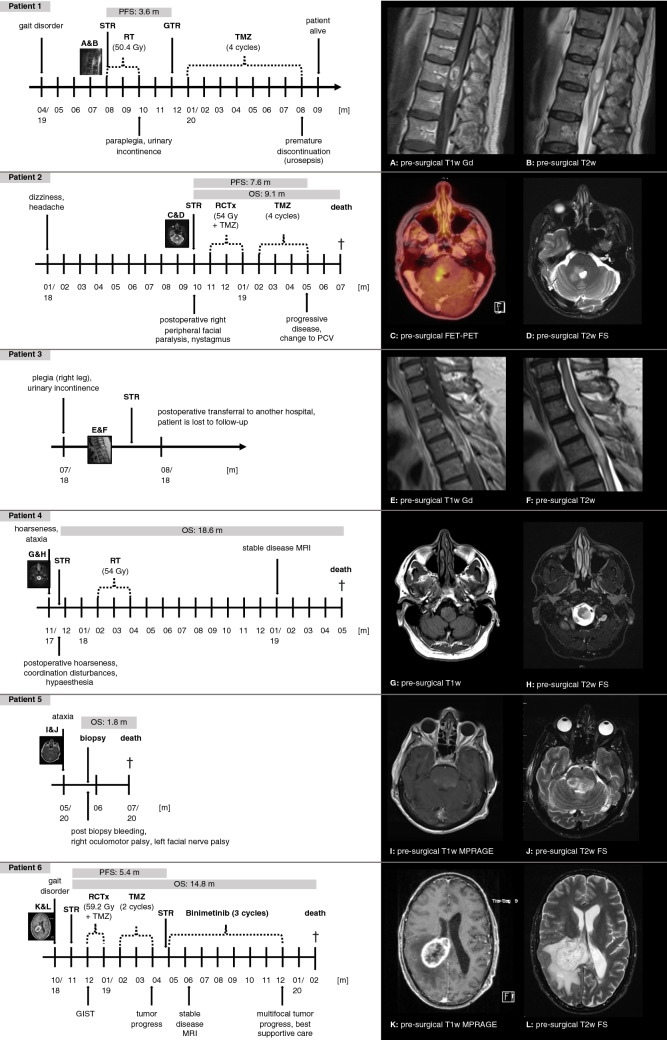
Clinical course of six patients with high-grade astrocytoma with piloid features including pre-operative MRI (A–L) and O-(2-[^18^F]fluoroethyl)-l-tyrosine positron emission tomography (FET-PET) (C). *FS* fat saturation, *Gd* Gadolinium, *Gy* Gray, *MPRAGE* magnetization prepared rapid gradient echo, *MRI* magnetic resonance imaging, *PCV* procarbazine, lomustine, and vincristine, *FET* O-(2-[^18^F]fluoroethyl)-l-tyrosine, *PET* positron emission tomography, *PFS* progression-free survival, *OS* overall survival, *RCTx* radiochemotherapy, *RT* radiotherapy, *STR* subtotal resection, *T* thoracic vertebra, *T1w* T1 weighted image, *T2w* T2 weighted image, *TMZ* temozolomide
